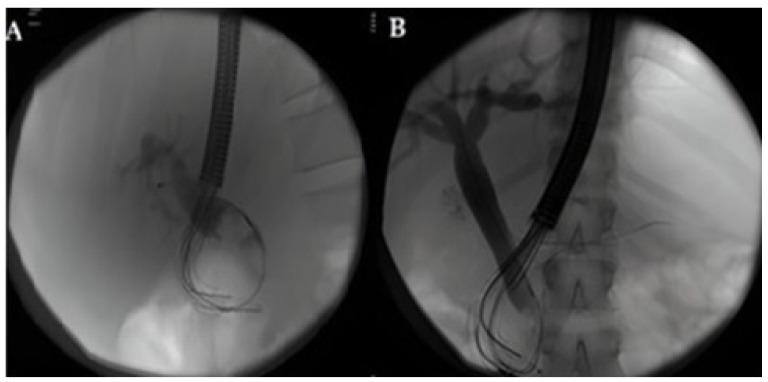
(A,B): X-ray image of an ERCP procedure with Ambu Single-Use Duodenoscope. Two different cases of elderly patients with cholangitis and many comorbidities. They had stones in the main bile duct that were successfully removed with basket.
